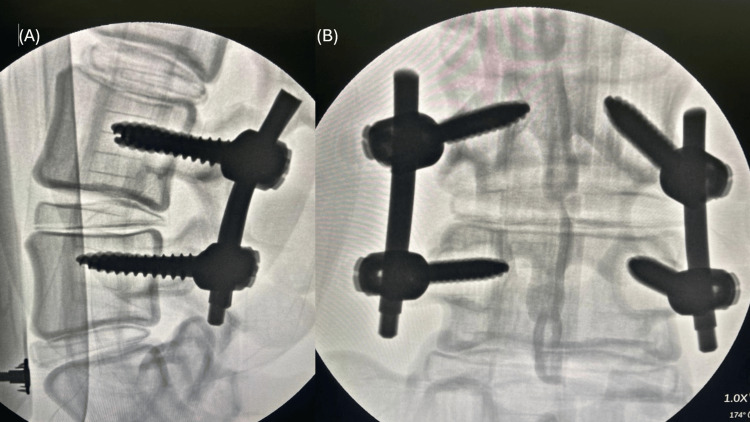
X-ray images of models with implanted screws, assessed for accuracy
